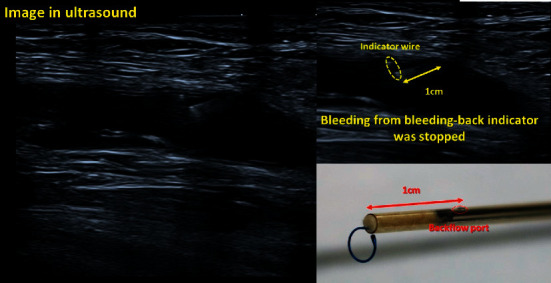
Ultrasound finding observed upon cessation of bleeding in the bleeding-back indicator.
